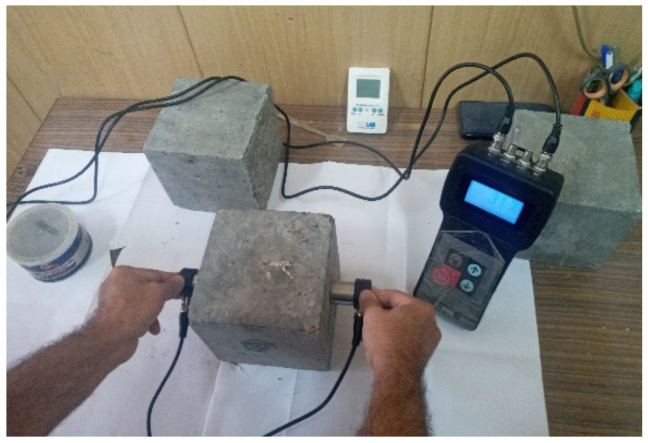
The ultrasound pulse velocity (UPV) tests.
